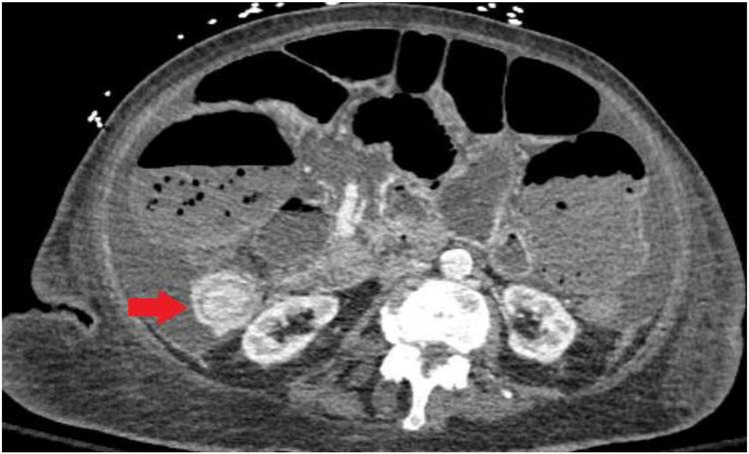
CT abdomen/pelvis displaying intussusception of terminal ileum.
